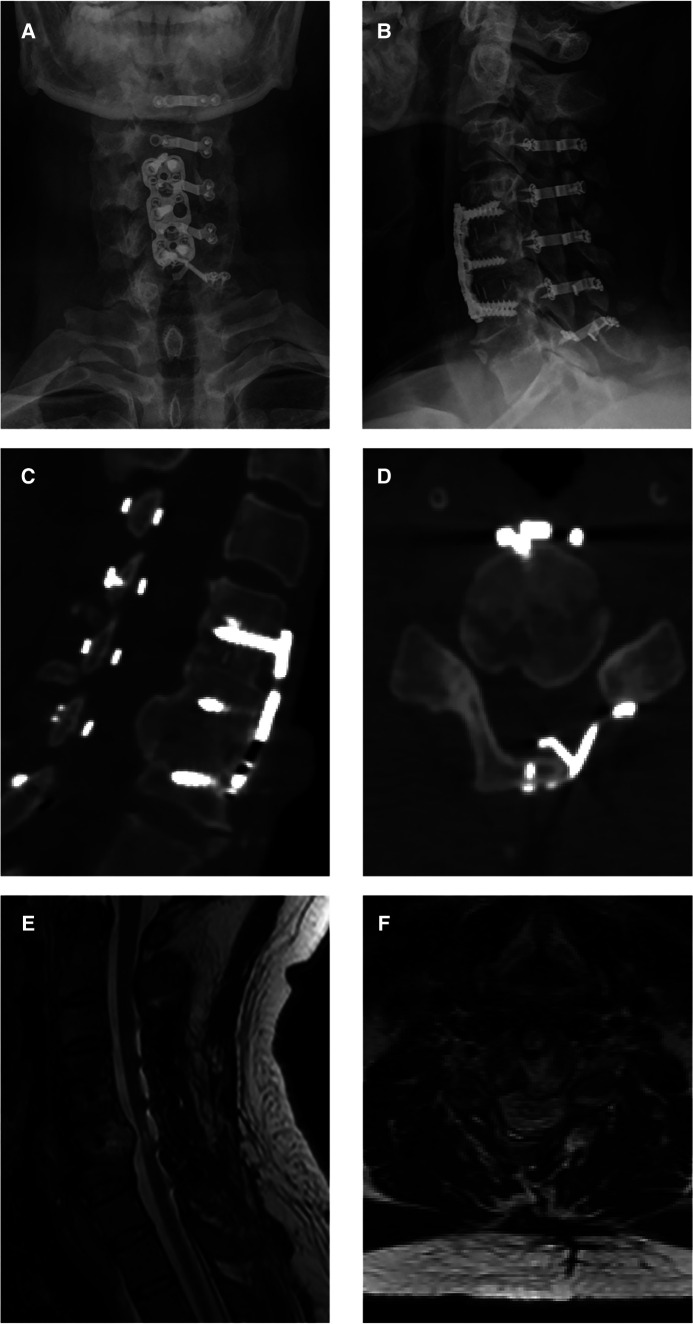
One-year follow-up images after open-door laminoplasty. (A,B) Postoperative x-ray images showing the accurate positions into which the plates and screws were installed. (C,D) Postoperative CT images showing direct adherence of all plates to the host bone, creating a new, wide canal space. (E,F) Postoperative MRI images showing decompression of the spinal cord at the C5/6 level.
